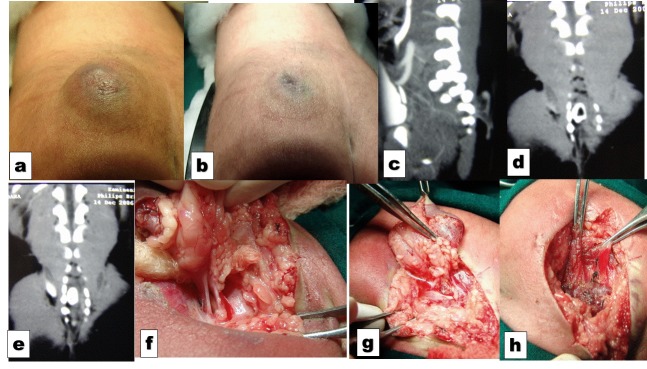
Figure 1: (a) Upper gluteal non tender soft cystic fluctuant reducible mass. (b) Collapsed on pressure. (c) Sagital view of CT scan showing the meningomyelocele. (d) Spindle shaped widening of interpeduncular space of sacrum with a defect in the bone laterally. (e) Coronal CT showing widened spinal canal with body fur. (f) Neural material seen with the margin of the defect. (g) Exteriorized neural placode in progress. (h) Gluteal flap to cover the dual defect that is closed underneath.
